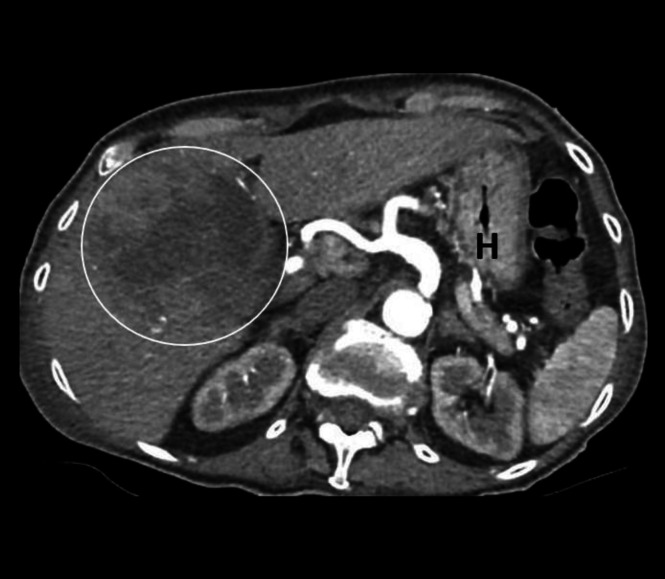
Contrast enhanced axial CT abdomen of a 90-year-old female with a history of hepatitis C infection, abdominal pain, abnormal liver function tests and elevated Alpha fetoprotein (AFP) shows a large, poorly defined lesion with areas of low attenuation (circled) in the right lobe of the liver. The lesion was subsequently diagnosed to be a necrotic hepatocellular carcinoma. Stomach (H) is collapsed and contains small amount of air. When collapsed, the wall of stomach appears thickened and should not be misinterpreted as pathological
